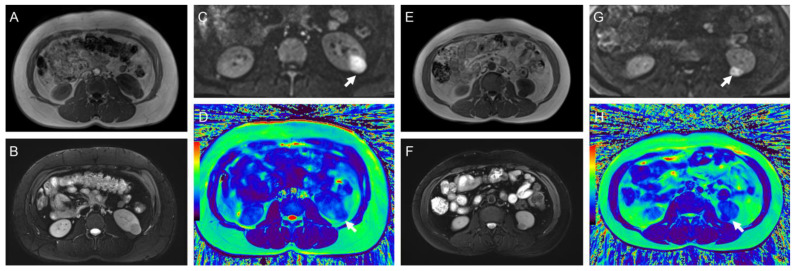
(A–D) Representative images of a 44-year-old woman with minimal fat angiomyolipoma in the left kidney (arrow): (A) axial T1-weighted image; (B) T2-weighted image; (C) diffusion-weighted images (b = 800 s/mm^2^); (D) radial TSE T2 map. The mean ADC and T2 values of the tumor were 0.69 mm^2^/s and 82.2 ms, respectively. (E–H) Representative images of a 44-year-old man with chromophobe RCC in the left kidney (arrow): (E) axial T1-weighted image; (F) T2-weighted image; (G) diffusion-weighted images (b = 800 s/mm^2^); (H) radial TSE T2 map. The mean ADC and T2 values of the tumor were 1.08 mm^2^/s and 116.0 ms, respectively. The color bar in the pseudocolor images (D and H) represents T2 values, which gradually increase from black to red.
